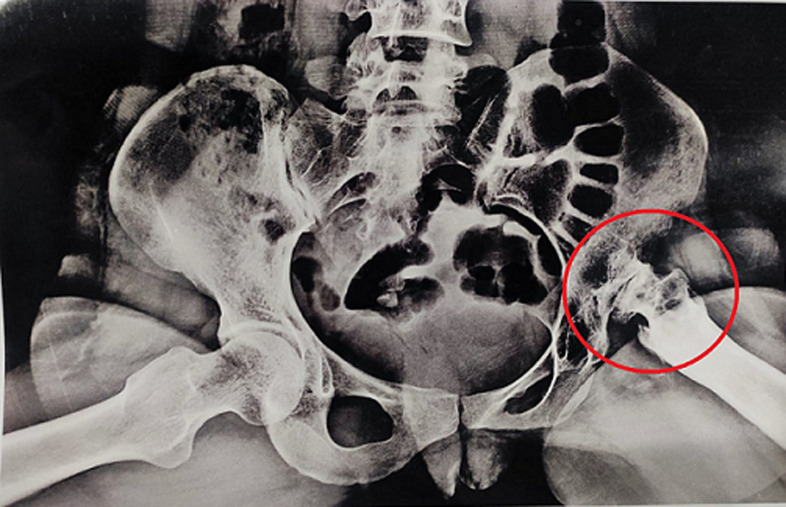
X-ray of pelvic showing grade IV avascular necrosis of left femoral head
